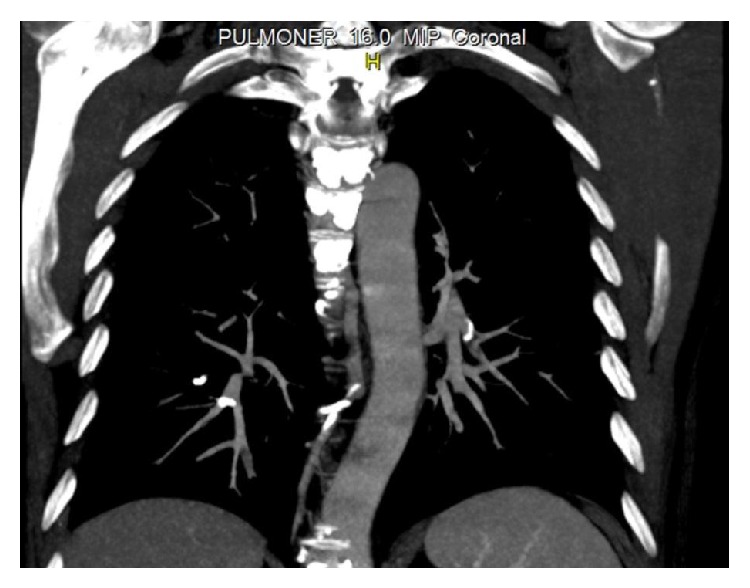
Coronal view of thorax CT.
